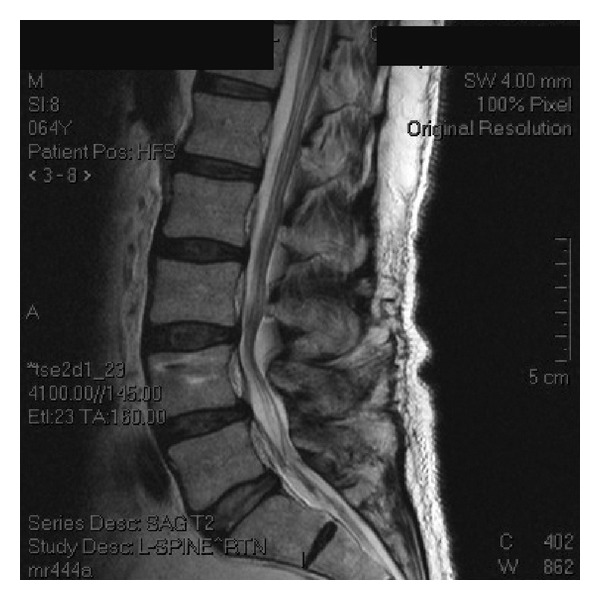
Midsagittal MRI at 2 years outdemonstrating maintenance of disc configuration at L4-5 and a well-hydrated supraadjacent segment.
